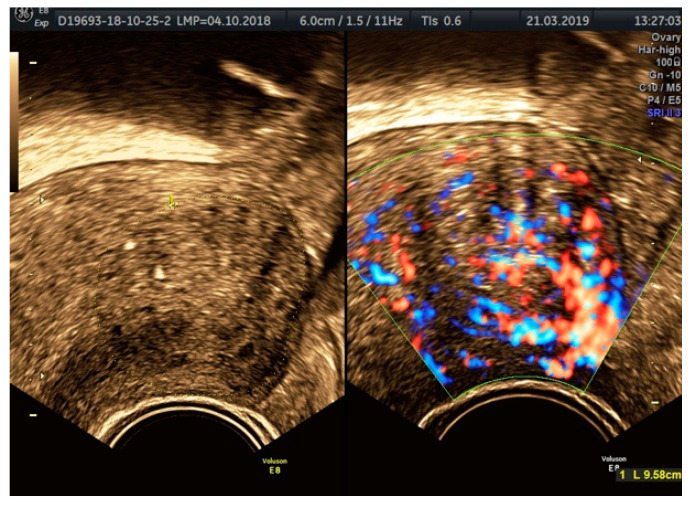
Ultrasound image of diffuse adenomyosis showing translesional blood flow in Doppler mode.
